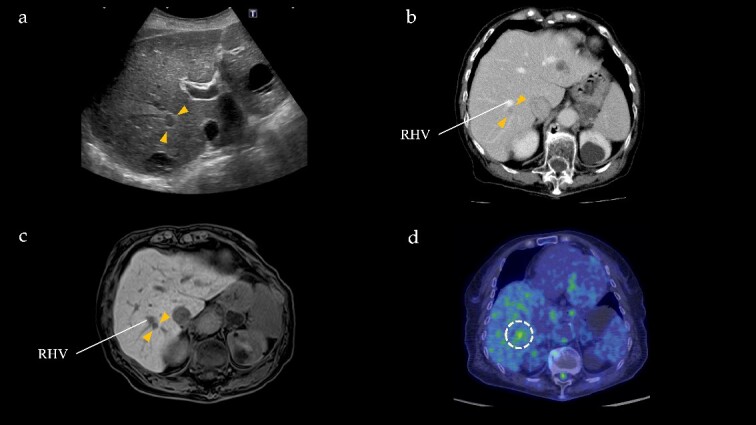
Radiographical findings of Segment 7 lesion in the liver. (a) On abdominal ultrasound, a hypoechoic tumor, measuring 10 mm (arrows), is identified in Segment 7. (b) Contrast-enhanced computed tomography indicates a 10 mm, hypodensity lesion located in Segment 7 (arrows) at portal phase. This lesion is close to the RHV. (c) Gadoxetic acid-enhanced magnetic resonance imaging shows a 10 mm, hypointensity lesion (arrows) in Segment 7 adjacent to the RHV at hepatic phase. (d) PET/CT reveals FDG accumulation (maximum standardized uptake value, SUVmax = 3.91) in the Segment 7 nodule.
